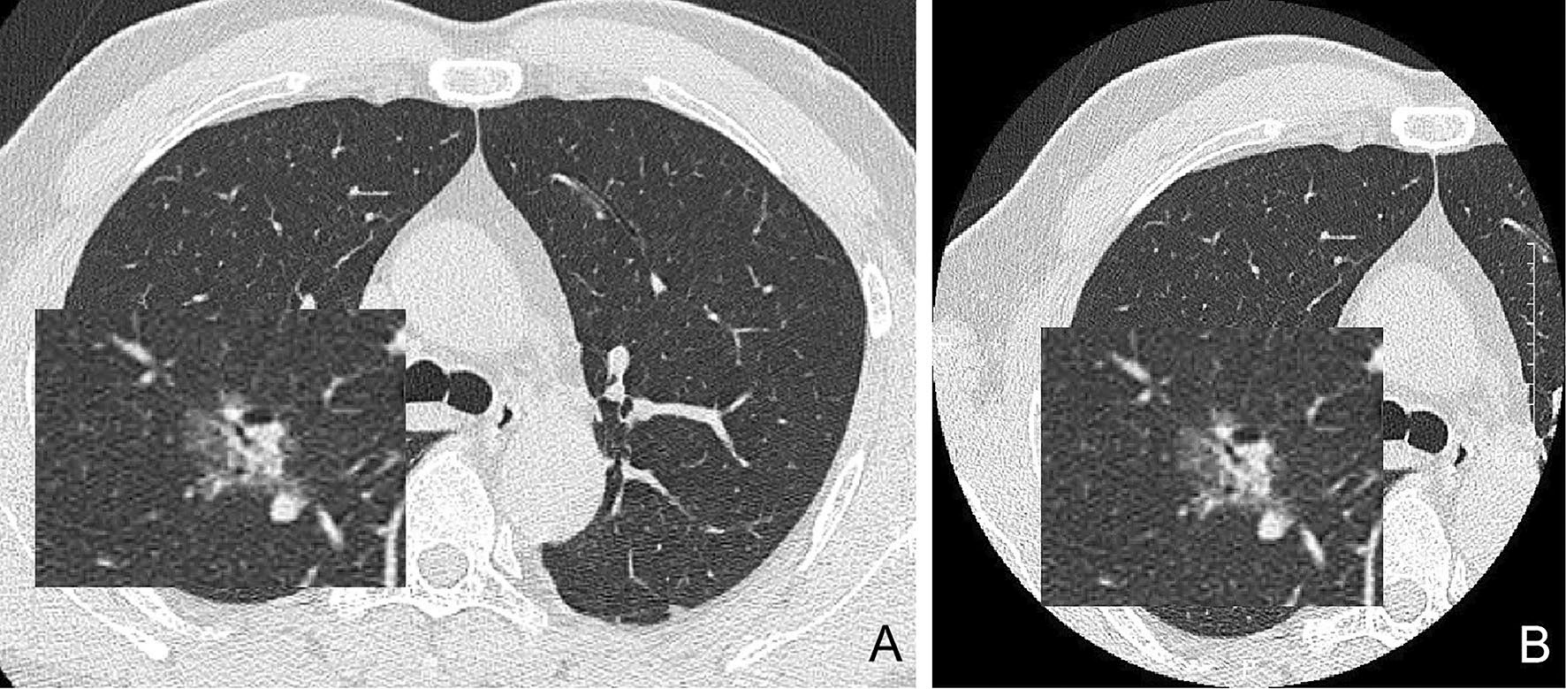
There was little difference between the c-CT and sFOV-CT images after subjective evaluation by radiologists.
